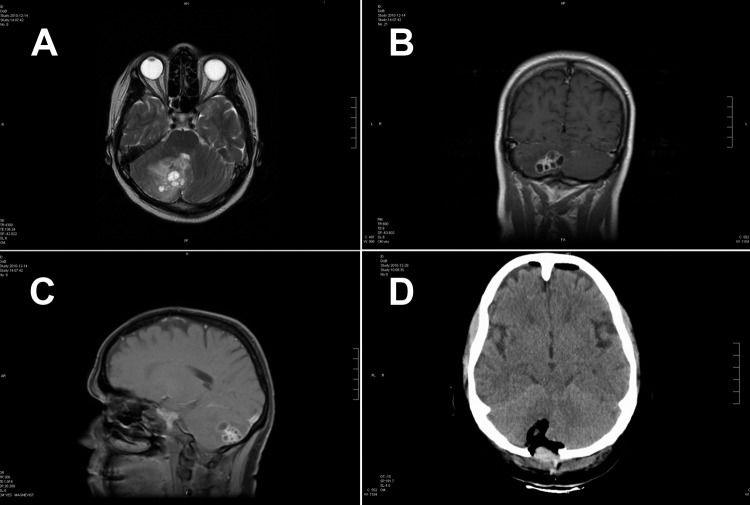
Magnetic resonance (MR) and computed tomographic images of the brain of a 51-year-old woman infected with *Taenia crassiceps* tapeworm larvae, Germany. A) Transverse view, T1-weighted MR image. The 30 × 30 mm parasitic lesion with perifocal edema is located in the right hemisphere of the cerebellum and caused ataxia, headache, and nausea. The fourth ventricle is compressed. B) Coronal view, T2-weighted MR image. The cyst-like appearance of the parasitic tissue is clearly visible. This lesion can be misinterpreted as cerebral echinococcosis, racemose cysticercosis caused by a *Taenia solium* tapeworm, or coenurosis. C) Sagittal view, MR image with contrast enhancing agent. D) Transverse view, computed tomographic image after surgery. No residual parasitic masses, only the parenchymal defect in the cerebellum after resection of *T*. *crassiceps* tapeworm larvae, are visible.
